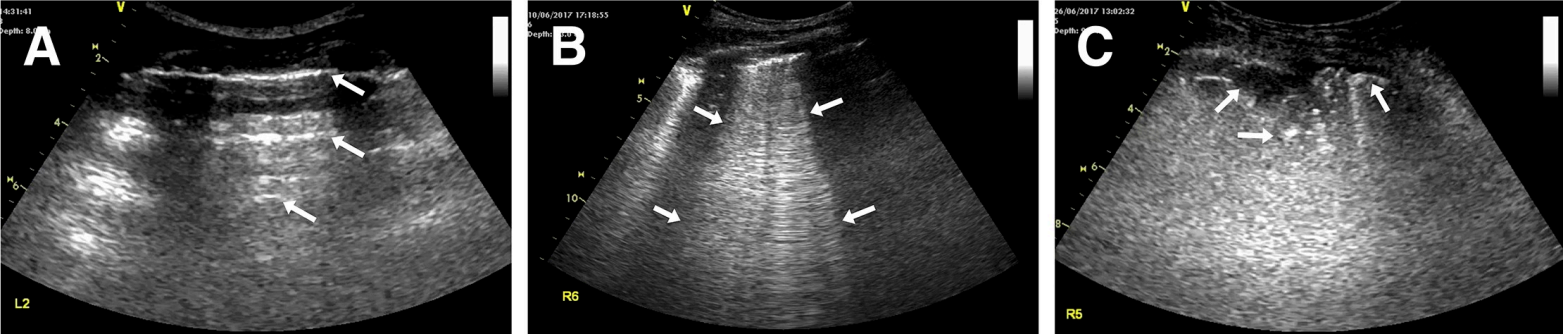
Common lung ultrasound patterns. (A) ‘A-lines’ are reverberation artefacts of the pleura line present in normally aerated lung tissue; they are indicated by the arrows. (B) ‘B-patterns’ are pathological signs of extravascular lung water. Here, multiple coalescent B-lines are shown between the indicator arrows, extending to the lower end of the screen. (C) ‘C-patterns’ are characteristic for pulmonary consolidation suggesting pneumonia. Here, an interruption of the normal pleural line is shown, with multiple echogenic punctiform laesions (as indicated by the arrows) and an underlying air bronchogram.
